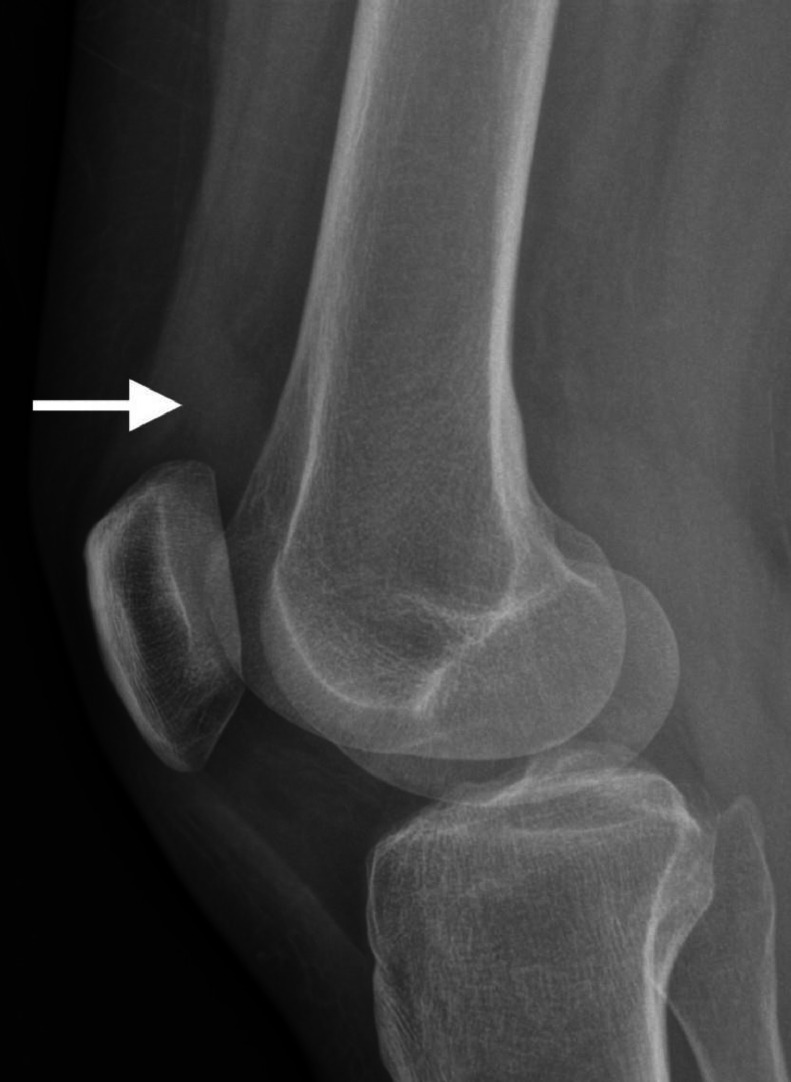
Lateral radiograph of the knee. There is a small suprapatellar knee effusion. No lipohaemarthrosis. No bony injury is identified.
